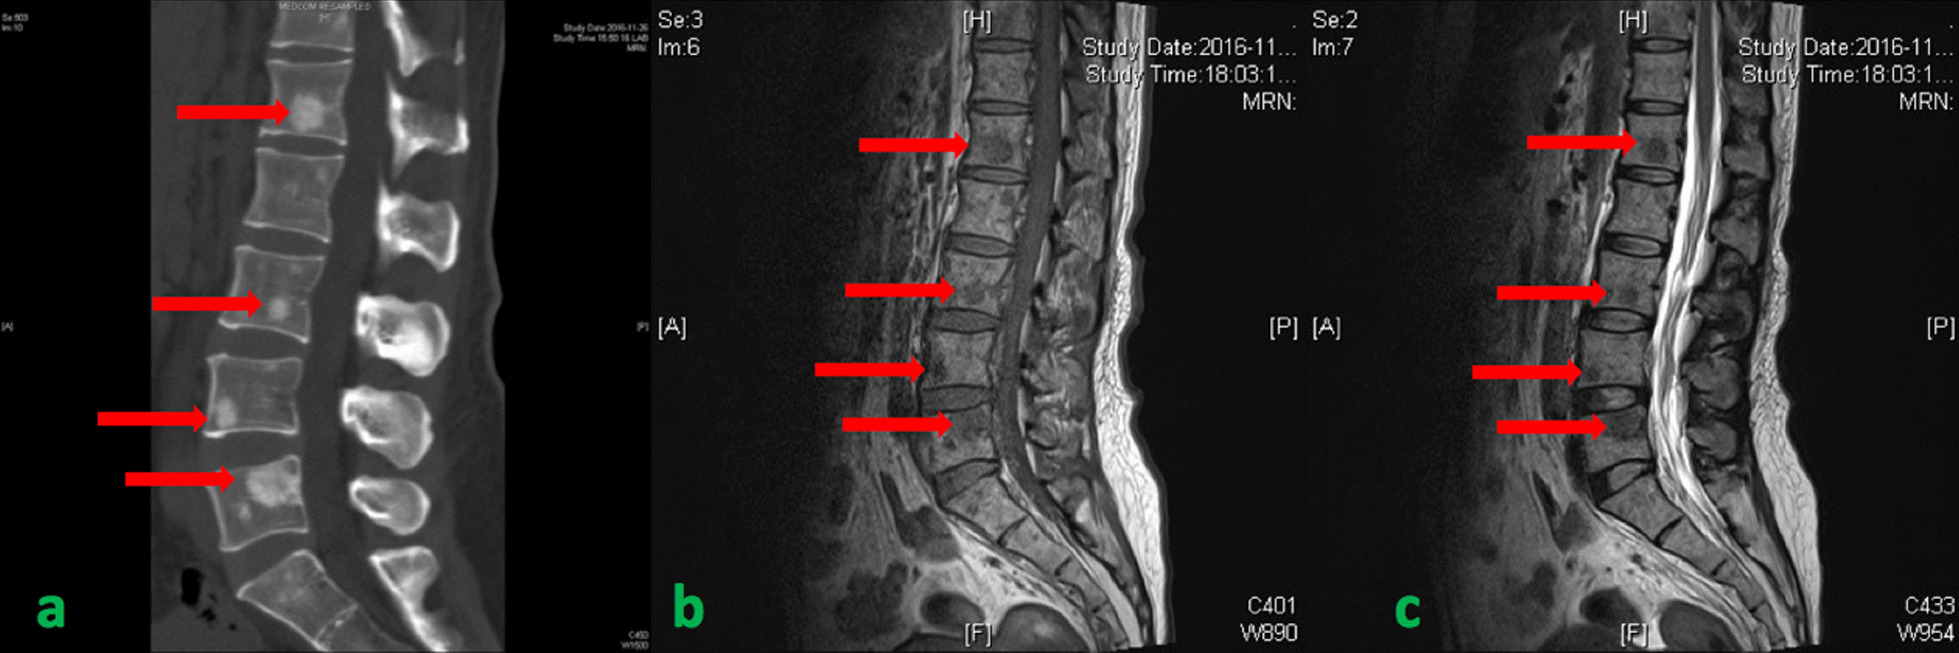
a CT scan showing multiple high-density lesions in lumbar vertebrae. b, c MRI demonstrating abnormal low signal in the corresponding vertebral bodies at T1 and T2 sequences. Red arrows point to the lesions
